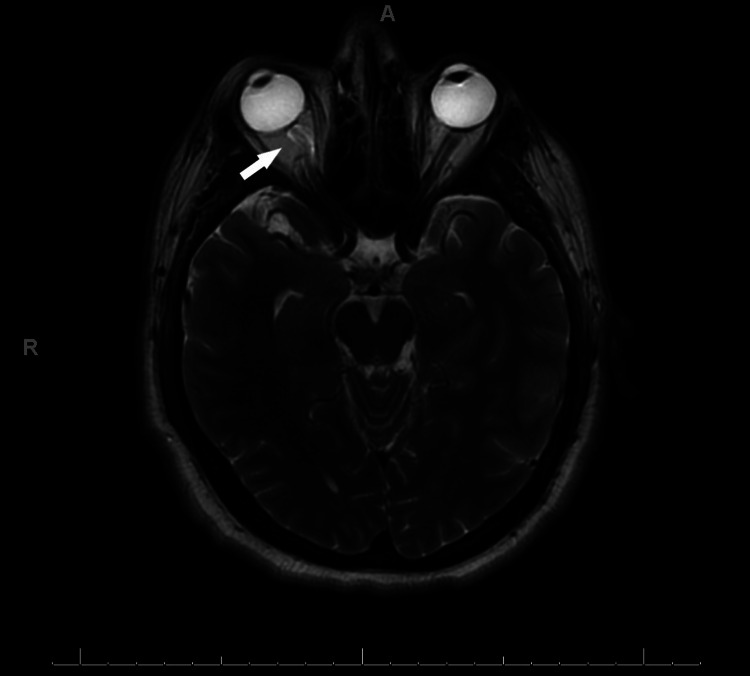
An MRI of the eye, orbit, and brain that is notable for a tortuous optic nerve sheath complex on the right (white arrow).
